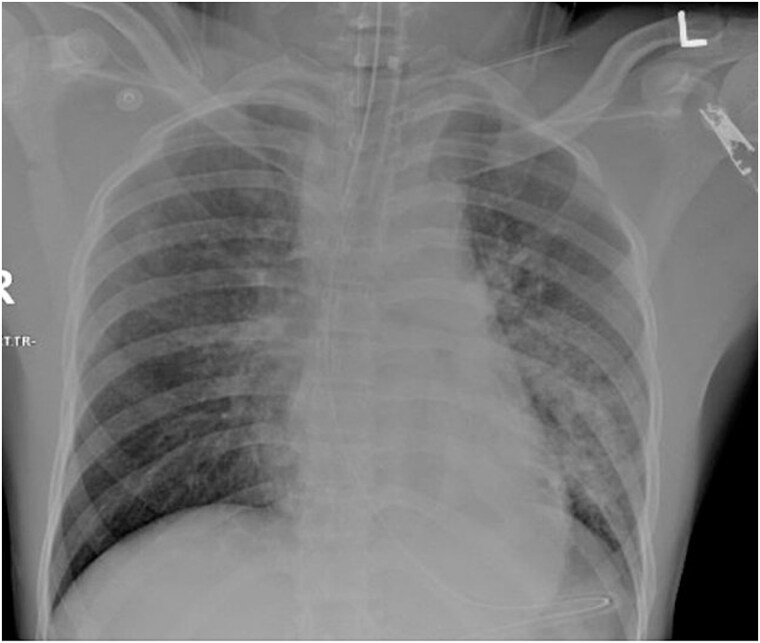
Initial chest X-ray showing widen mediastinum.
